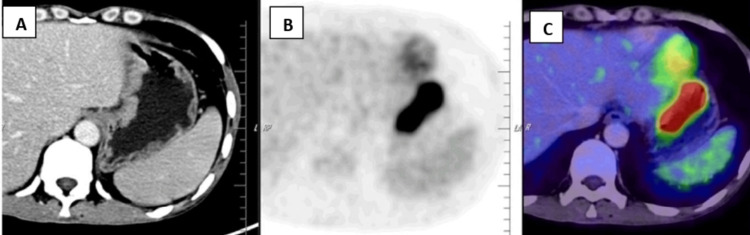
FDG PET scan showing avid, ill-defined wall thickening along the lesser curvature of the cardia, body, and adjacent antrum of the stomach, with suspicious periserosal infiltration and loss of fat plane with the left lobe of the liver (A) Irregular wall thickening along the lesser curvature of the stomach. (B) Increased radiotracer uptake. (C) Metabolically active lesion along the lesser curvature of the stomach, SUV max 23. FDG, fluorodeoxyglucose
